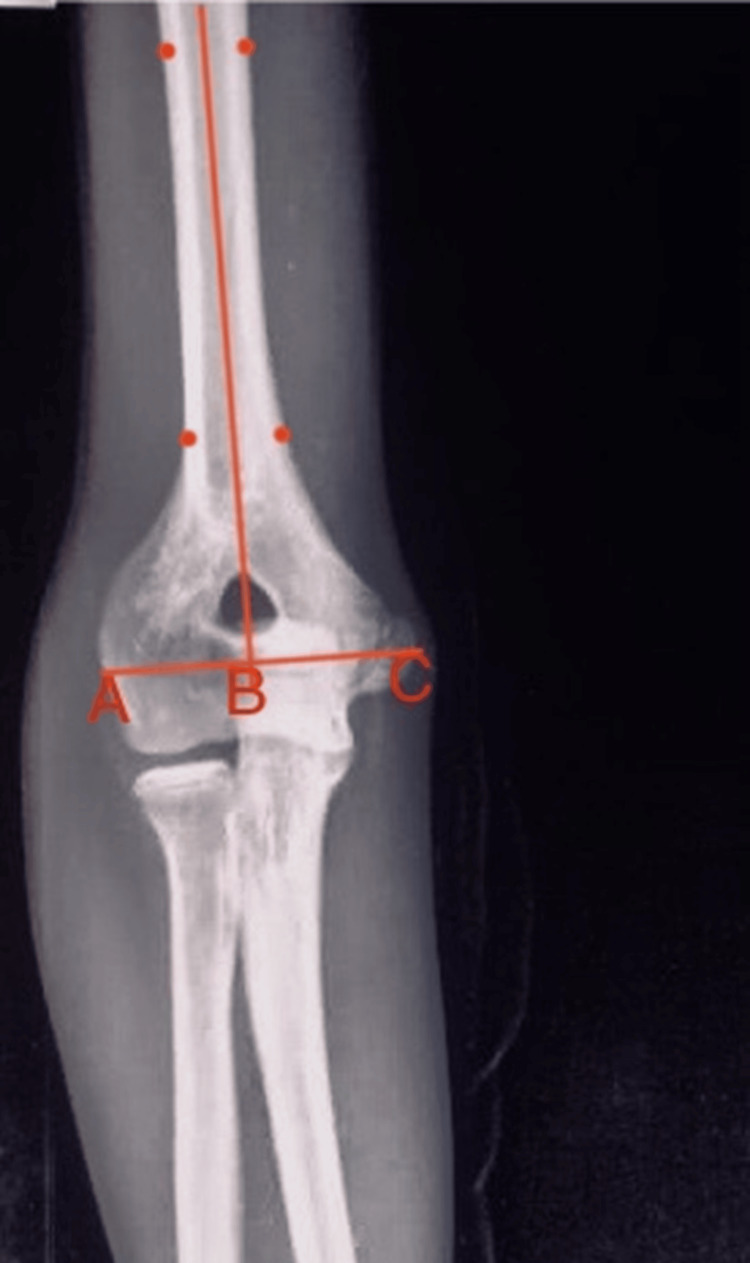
The LCPI calculated from AP elbow radiographs LCPI: Lateral condylar prominence index, AP: Anteroposterior
